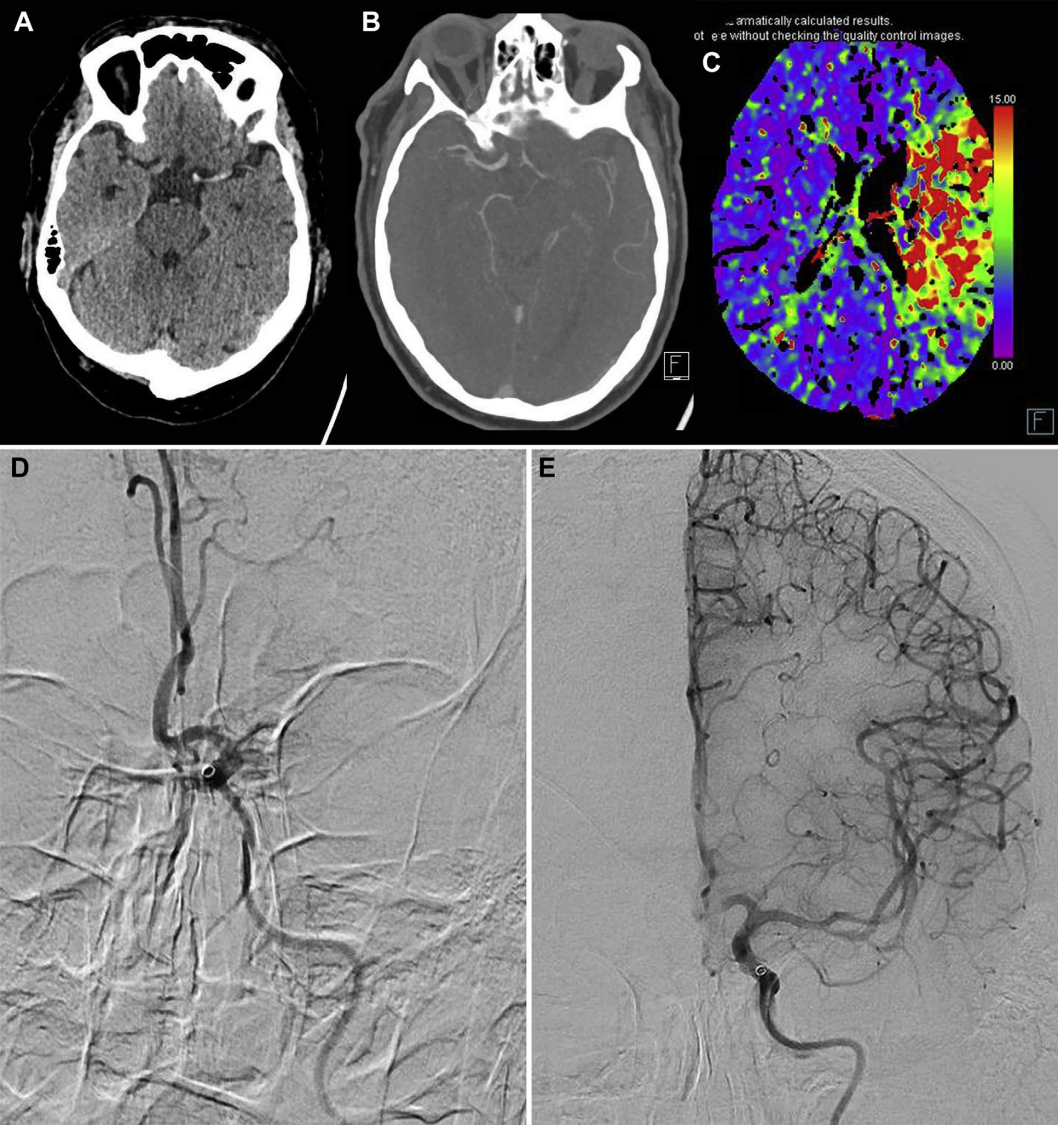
A 62-year-old COVID-19-positive man presented with right-sided hemiparesis. A-C, Cerebral computed tomography showed (A) a left dense media sign, (B) occlusion on computed tomography angiography (CTA), and (C) corresponding perfusion defect. D, Digital subtraction angiography confirmed an M1 occlusion. E, After intra-arterial thrombectomy, cerebral blood flow was restored (Thrombolysis in Cerebral Infarction grade 2C).
